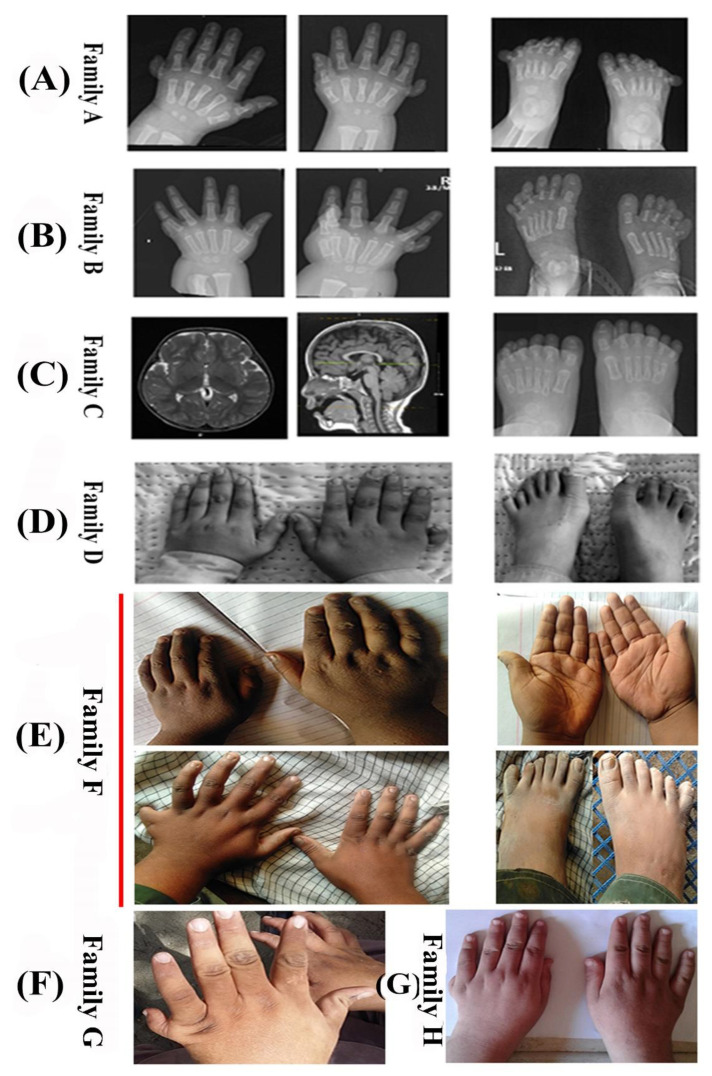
(A–G) Radiographs and pictures of hands and feet of the affected individuals in families (A–D) and (F–H).
